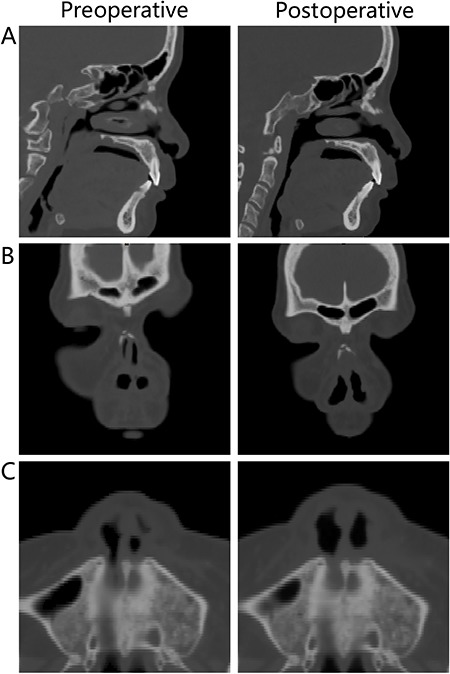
Pre- and postoperative anterior nasal cavity stenosis. *A*, sagittal and *B*, coronal computed tomography of the nasal cavity. *C*, Nasal base view.
